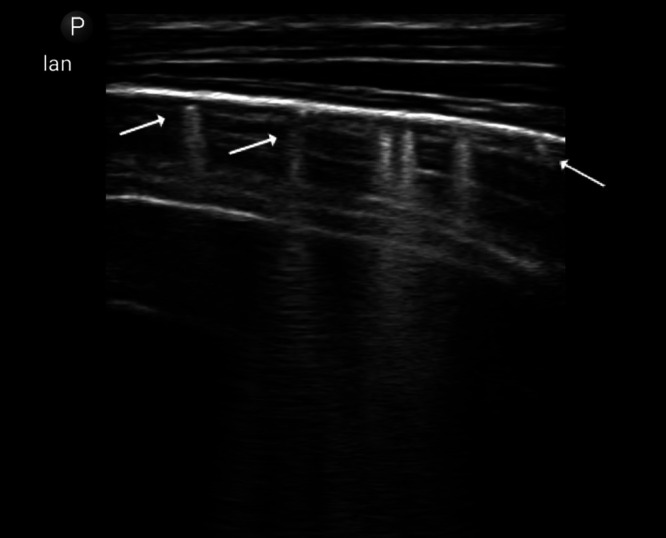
Sonographic miliary pattern, characterized by B-lines (arrowhead) and subpleural granularity (thin arrows) in almost every scanning zone, best seen during respiratory movement (Supplementary Video 1). This 40-year-old HIV-positive patient presented with 2 months of fever, cough, and weight loss and was unable to produce sputum for acid-fast bacillus testing. Abdominal and cardiac imaging showed splenic microabscesses and a small pericardial effusion, further supporting a diagnosis of disseminated tuberculosis. The patient was started on treatment for tuberculosis and adult malnutrition and discharged home with gradual improvement after 10 days.
